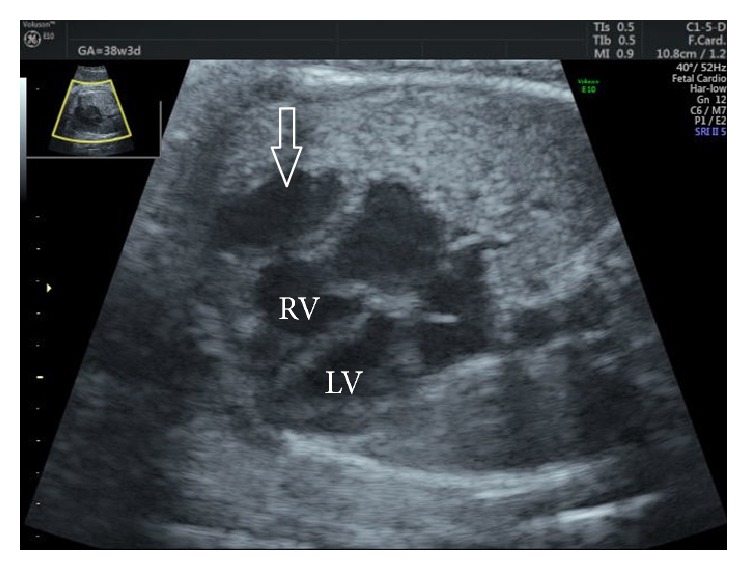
Fetal ultrasound: four-chamber view showing the diverticulum arising from the right ventricle. LV, left ventricle; RV, right ventricle.
